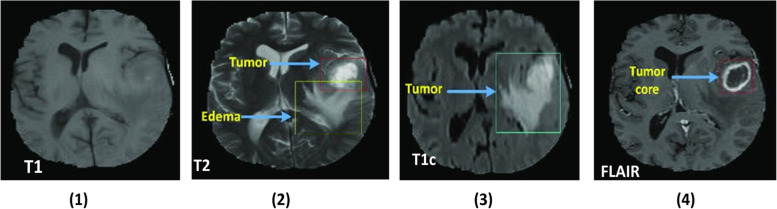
Brain Tumor Images in BraTS2020 (1) for Type T1, (2) for Tumor Type T2, (3) for Tumor Type T1c, and (4) for Tumor type FLAIR
